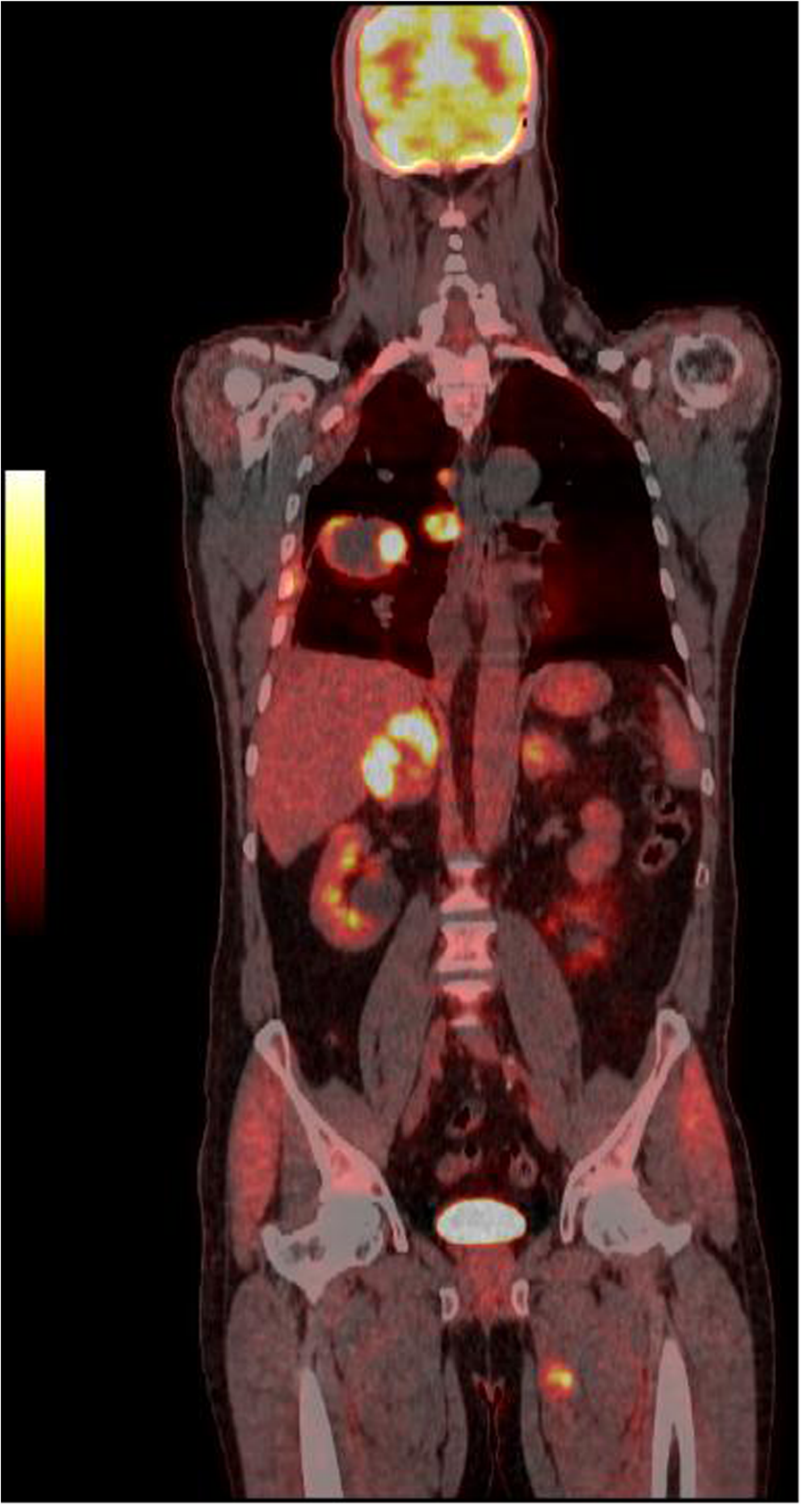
Coronal 18F-fluorodeoxyglucose positron emission tomography/computed tomography fused image showing hypermetabolic foci in the right lung, mediastinal lymph node, adrenal glands and the left thigh
